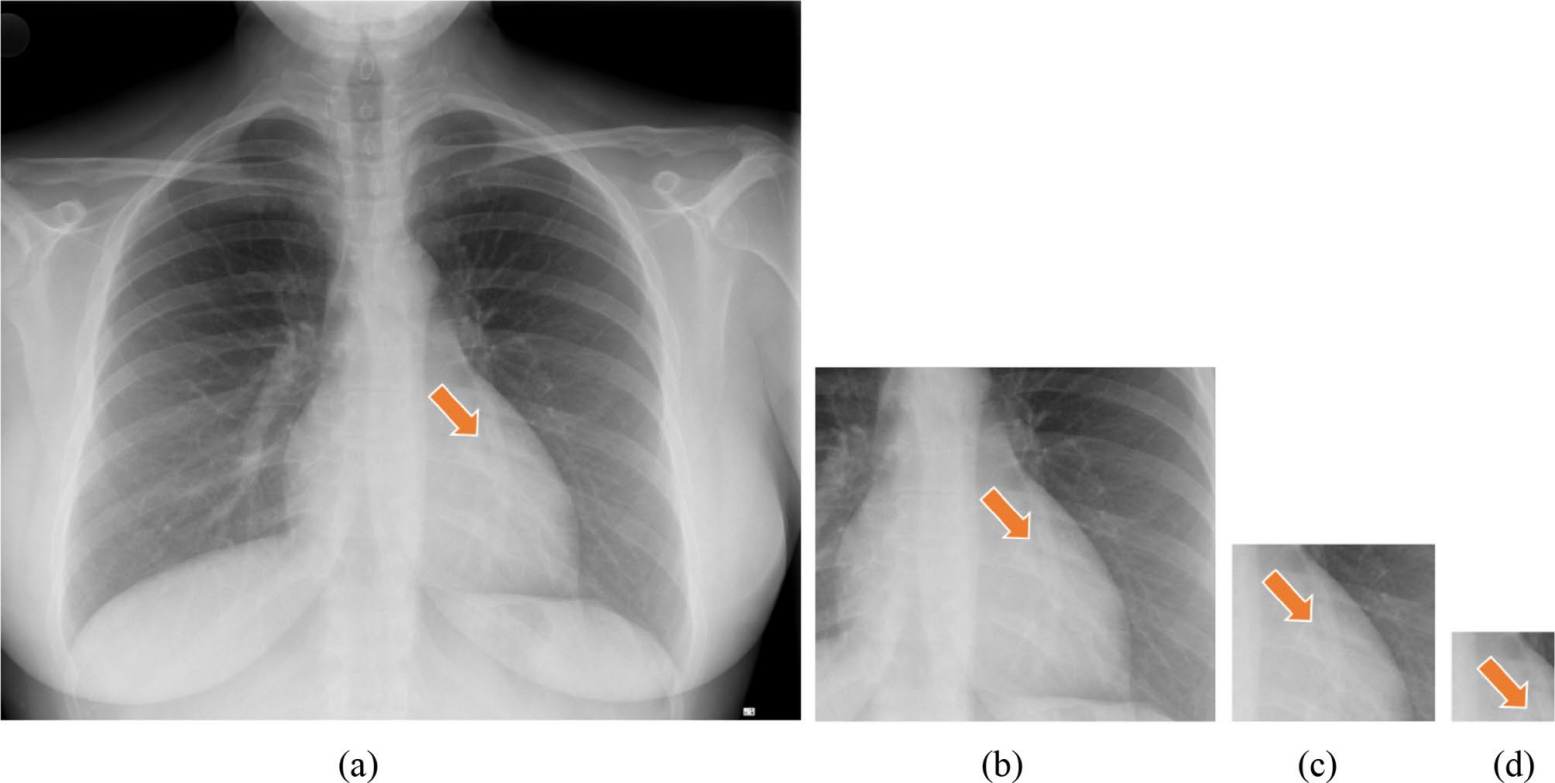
(a) Effect of patch resolution on identifying radiographic findings. An input CXR image of size W × H is divided into patch images of different sizes. (b–d) Examples of resulting patch images of sizes (b) W/2 × H/2, (c) W/4 × H/4, and (d) W/8 × H/8. The arrows identify the location of the patch images within the original input CXR image.
